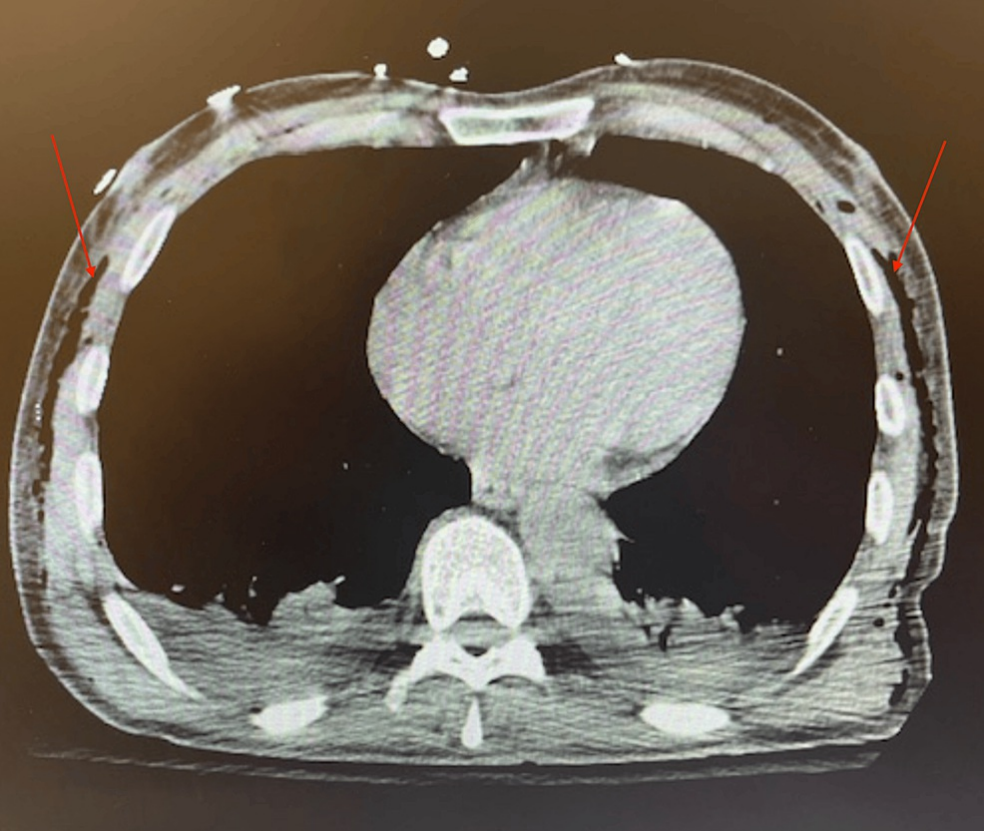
Repeat CT scan of the chest taken on postoperative day 6, which demonstrated marked improvement in subcutaneous emphysema with mild bilateral pleural effusions and bibasilar subsegmental atelectasis.
